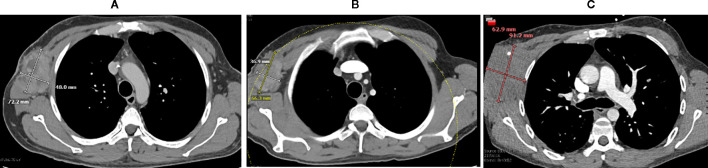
Right axillary lesion and radiographic responses to TIL. (A) CT chest obtained prior to TIL infusion (September 2024) demonstrating the right axillary mass measuring 72.2 mm × 48 mm. (B) CT chest obtained two months after TIL therapy (December 2024) demonstrating interval decrease in lesion size to 66.3 mm × 36.9 mm. (C) CT chest obtained five months after TIL therapy (February 2025) demonstrating interval enlargement of the lesion to 91.2 mm × 62.9 mm. These images correspond to the representative axillary mass shown in Image 1.
